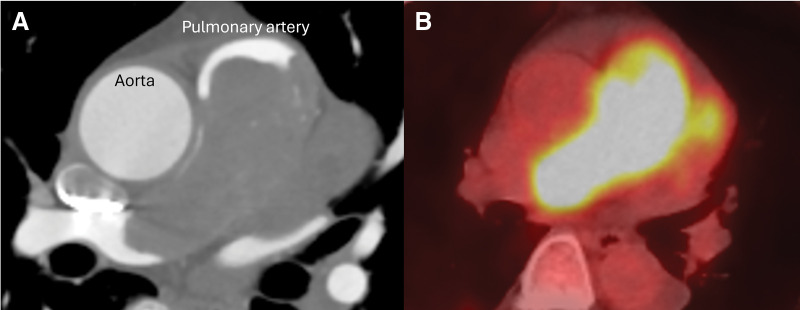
Preoperative (A) contrast-enhanced computed tomography showed a tumor embolizing the pulmonary artery trunk, involving the right ventricular wall and interventricular septum, and (B) fluorine-18 fluorodeoxyglucose positron emission tomography showed glucose activity at the pulmonary artery mass with a maximum standardized uptake value of 13.0, which is suggestive of a malignant cardiac tumor.
